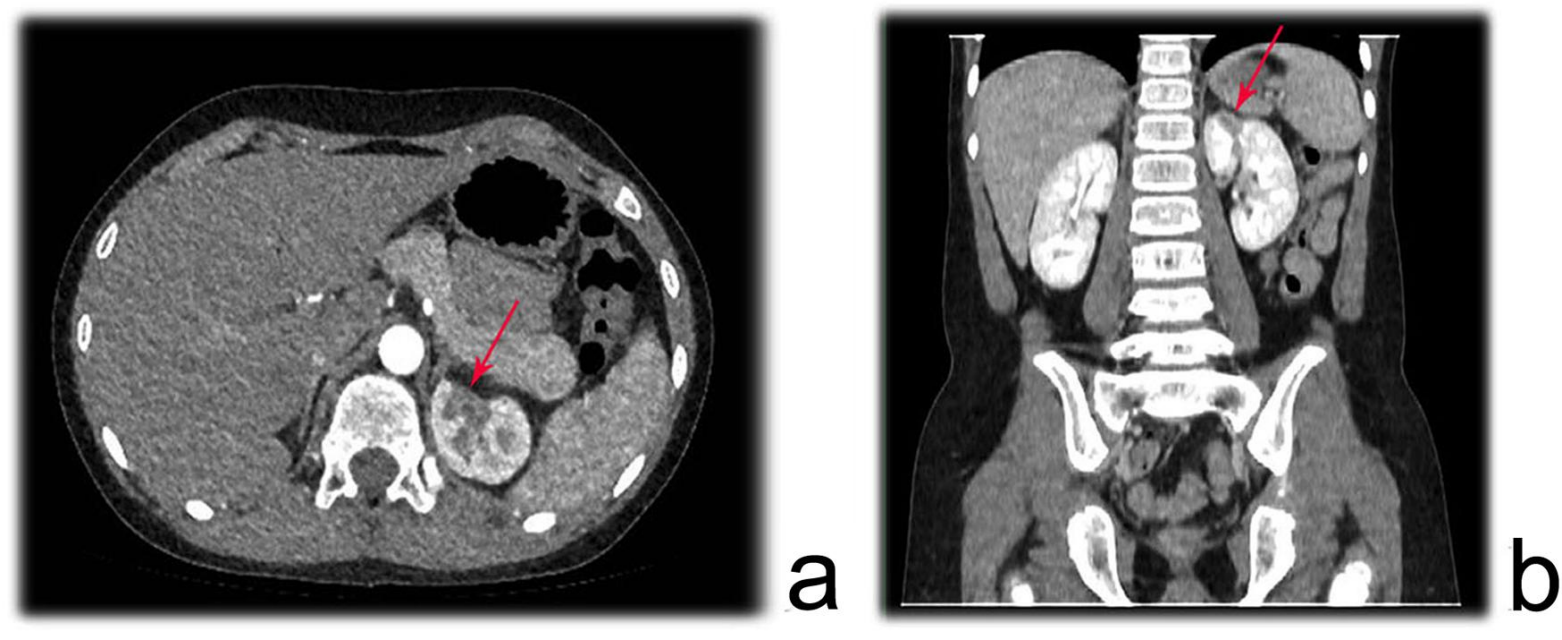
Renal CTA showing a region of reduced perfusion in the upper pole of the left kidney. (a) axial plane; (b) Coronal plane.
